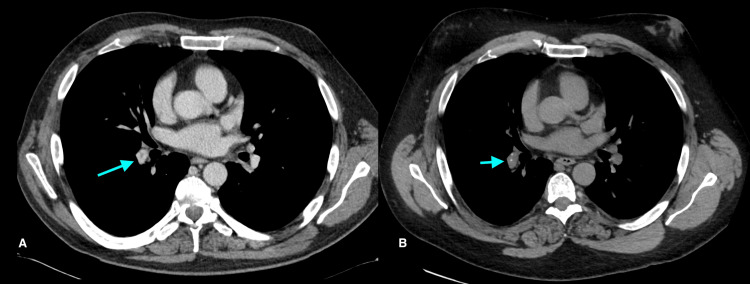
Chronic pulmonary embolus on initial CT (long arrow). B. Calcification of the chronic thrombus four years later (short arrow). A. Chronic pulmonary embolus in the right interlobar pulmonary artery on initial presenting CT in 2017 (left, long arrow). B. Calcification of the chronic thrombus developed in on follow-up CT four years later (right, short arrow).
